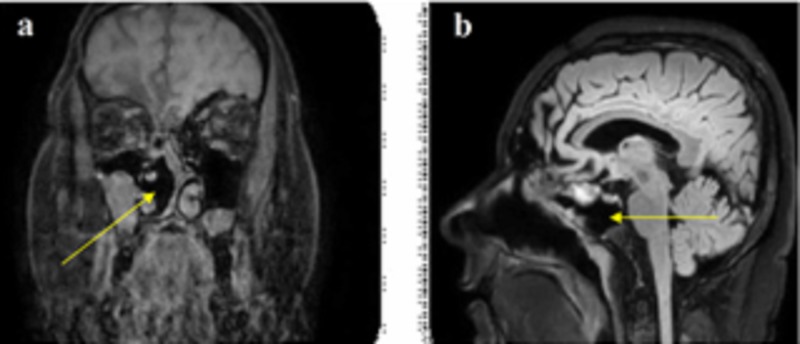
MRI brain scan [coronal (a) and sagittal (b) view] one year postoperatively demonstrating no recurrence of meningoencephalocele into the right nasal cavity (yellow arrow) and no evidence of CSF.
